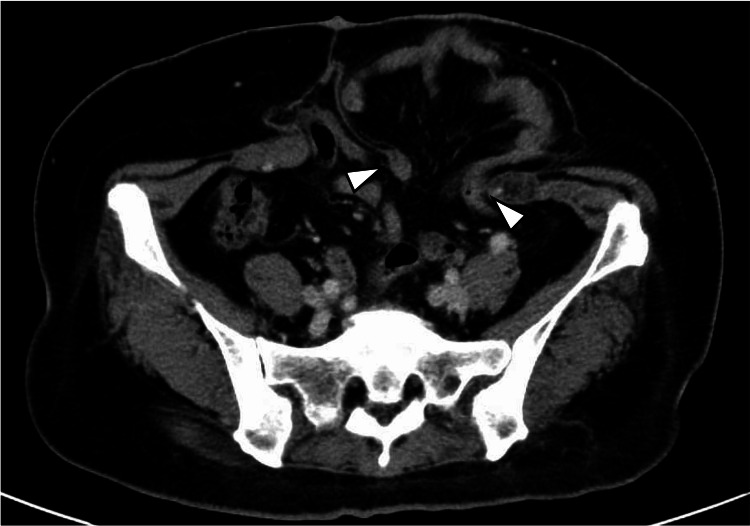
Parastomal hernia Contrast-enhanced computed tomography revealed a parastomal hernia with an orifice of 8.0x4.5 cm. Small intestinal prolapses were evident in the hernia (arrowheads).
